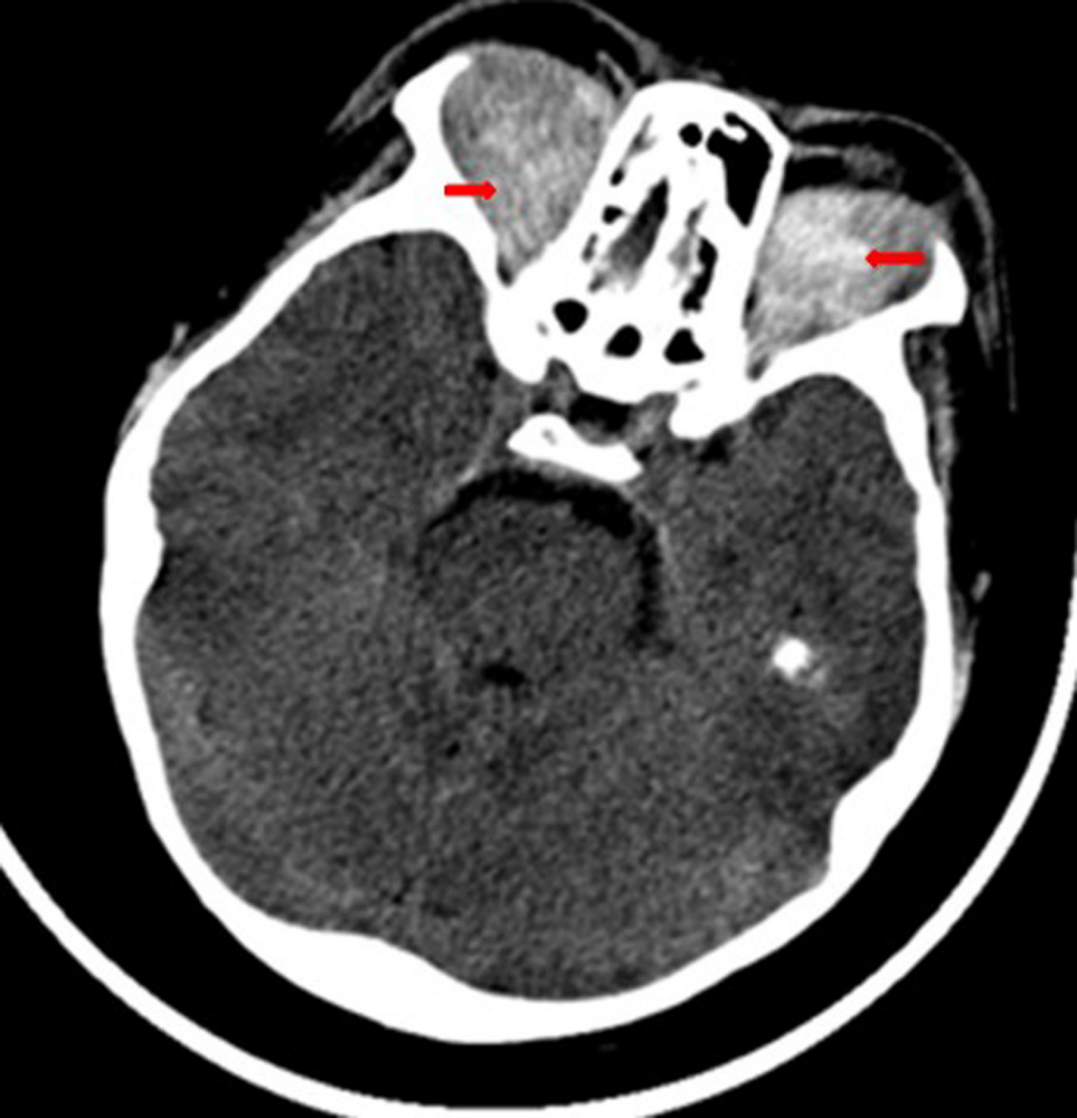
Oculocerebral CT scan with parenchymal window: bilateral intraorbital formation that is spontaneously hyperdense, consisting of both intra‐ and extraconal compartments, and of hematic density
